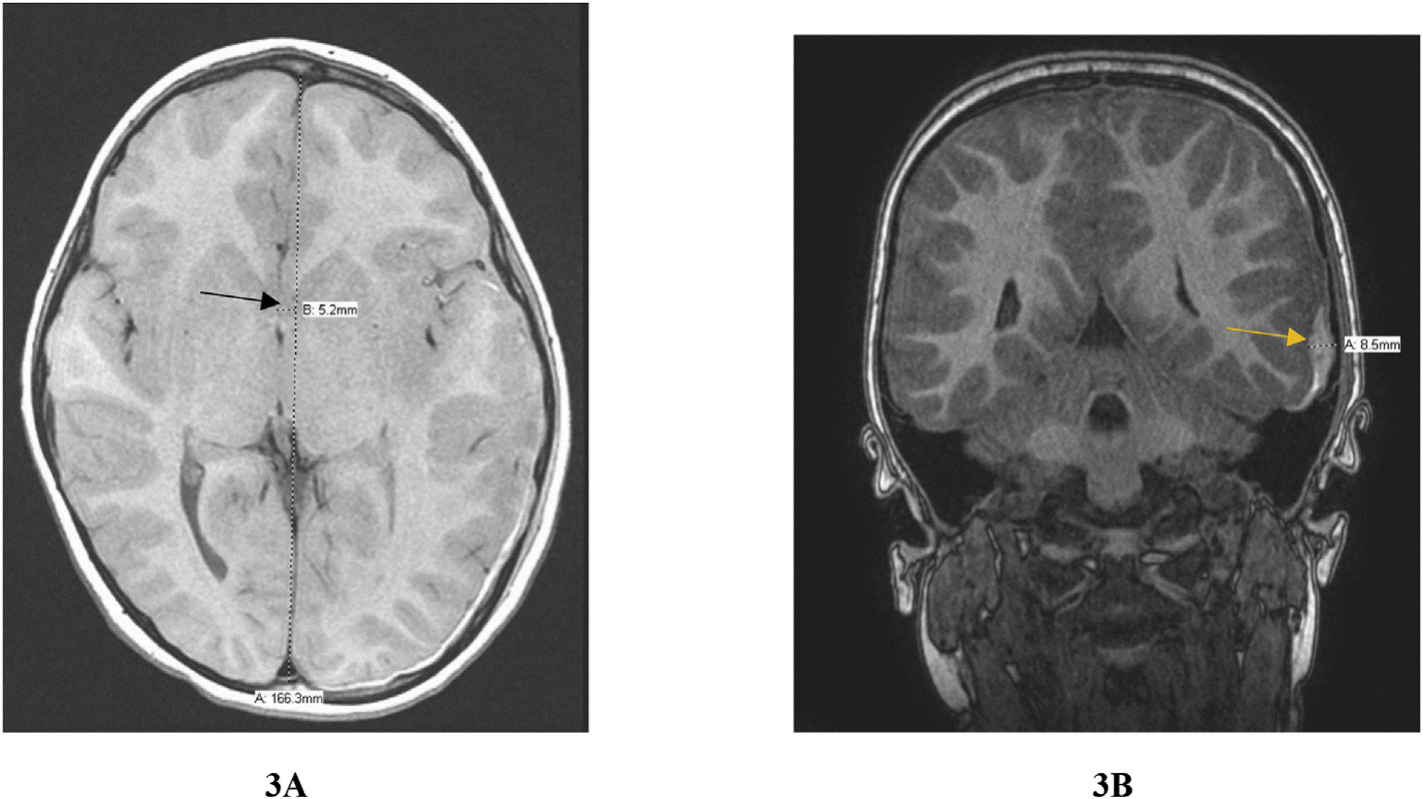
MRI scan completed on second day of hospital admission after bradycardic episodes. Image 3A highlights the midline shift (5.2mm) and Image 3B shows the expanding hematoma (8.5mm).
